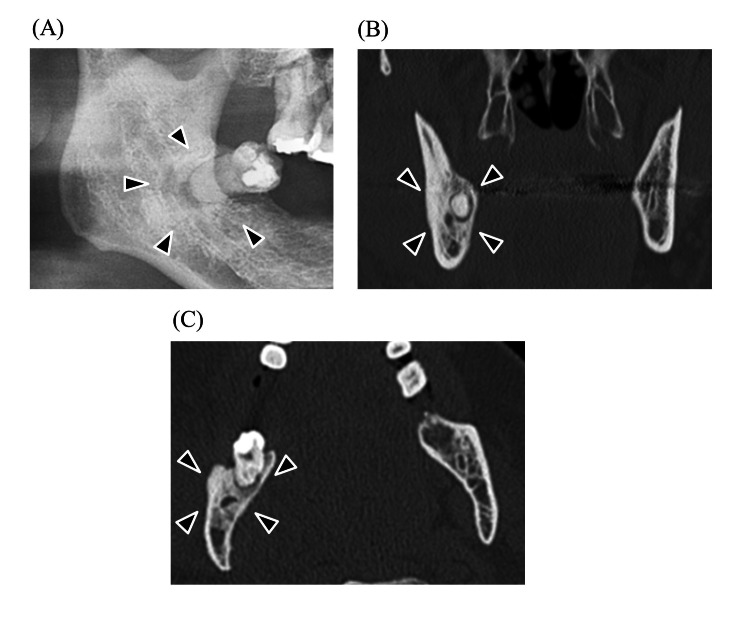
Radiopaque change in bone surrounding tooth (A) Panoramic image, (B) coronal CT image, and (C) axial CT image. Black arrows indicate the part of a radiopaque change in bone surrounding tooth.
